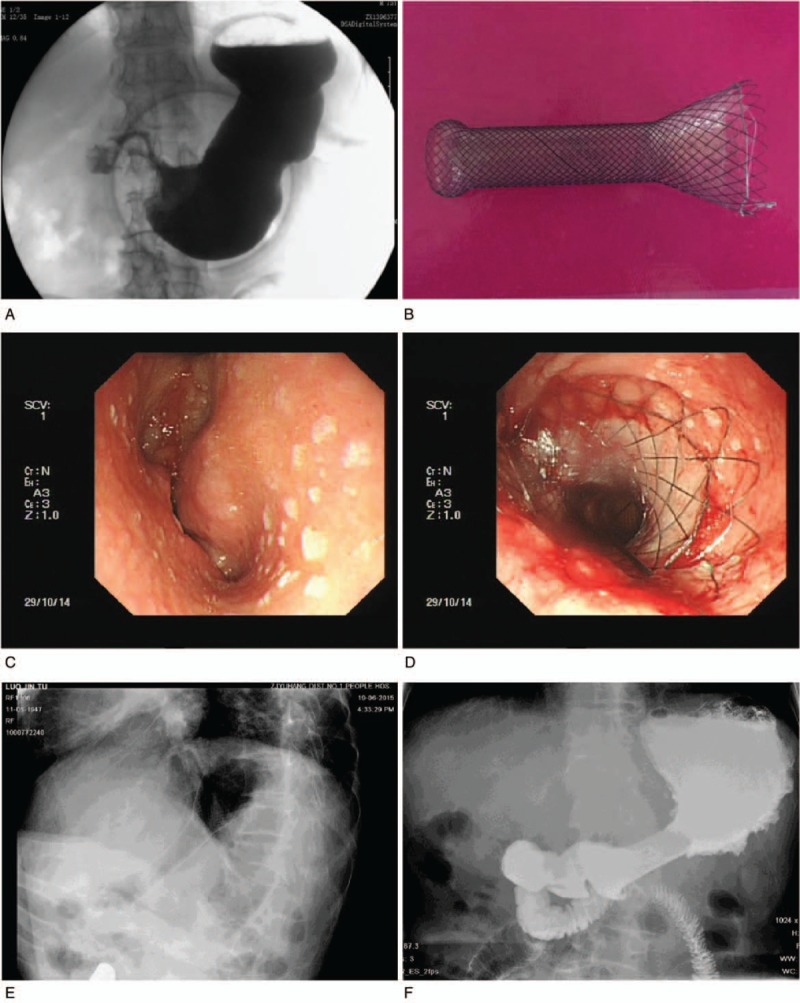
Use of a partially covered funnel stent in treatment of funnel shaped GOO. A, A 4 cm funnel shaped obstruction without contrast in duodenum. B, Model of funnel stent used to resolve the obstruction. C, Endoscopic view of the gastric tumor. D, Endoscopic view of the proximal funnel stent at the pyloric area. E, Confirmation of stent deployment by fluoroscopy. D, Barium contrast radiography shows contrast agent filling the stent. GOO = gastric outlet obstruction.
